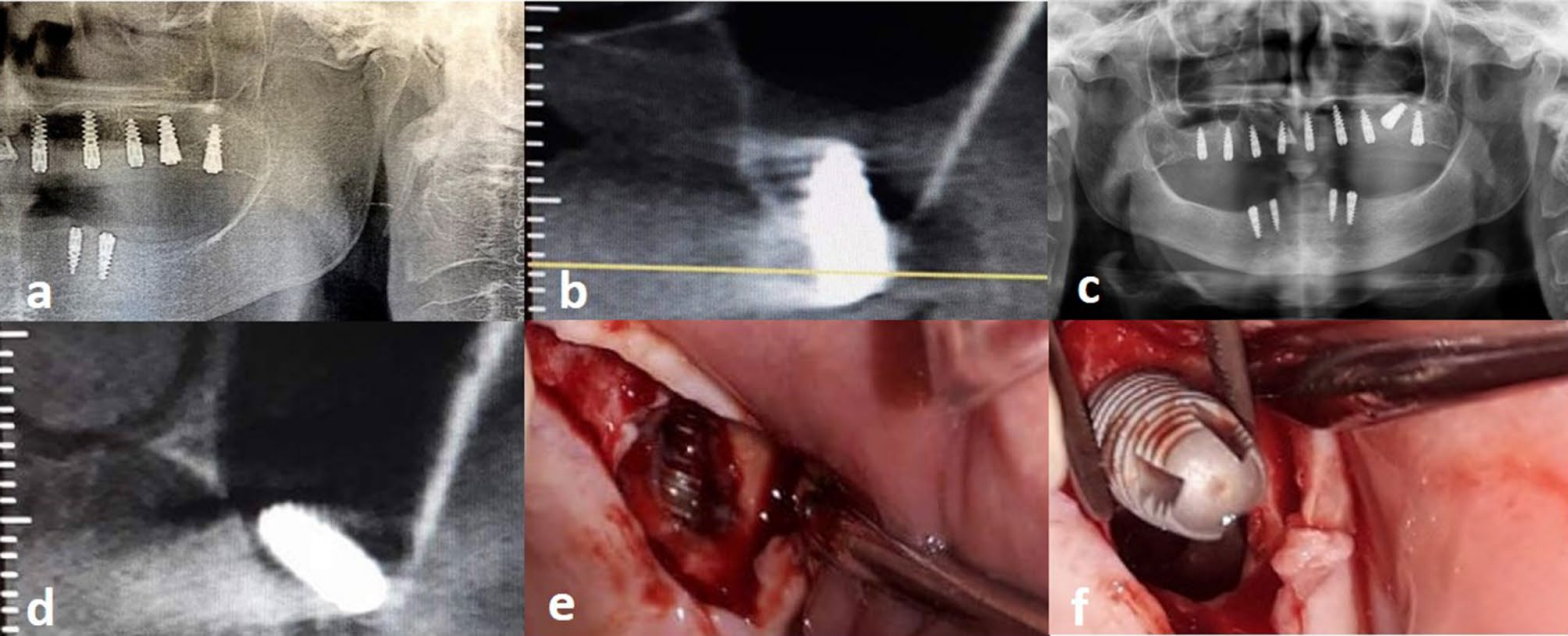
Two dimentional  X-ray exhibiting a limited vertical residual bone in the upper posterior maxillary area (a); immediate coronal CBCT showing implant surrounded by bone graft (b); implant migration three weeks after crestal sinus lifting (c,d); envelope crestal incision, surgical window in the alveolar bone followed by implant catching and retrieval through transalvoelar approach (e,f).
